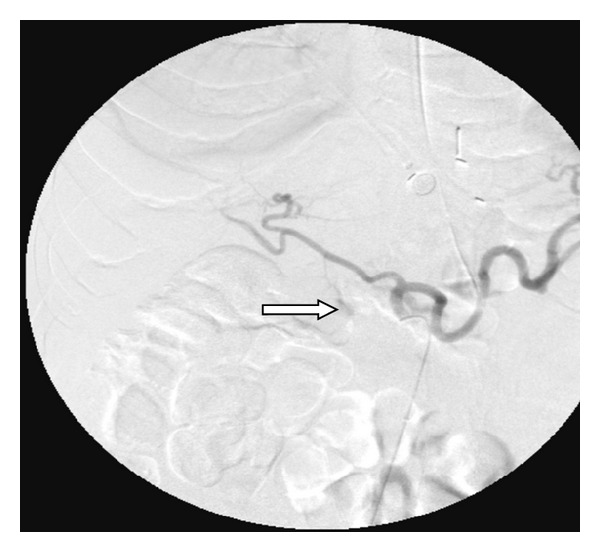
X-ray angiogram of the abdomen revealed pseudoaneurysms in the distal gastroduodenal artery.
